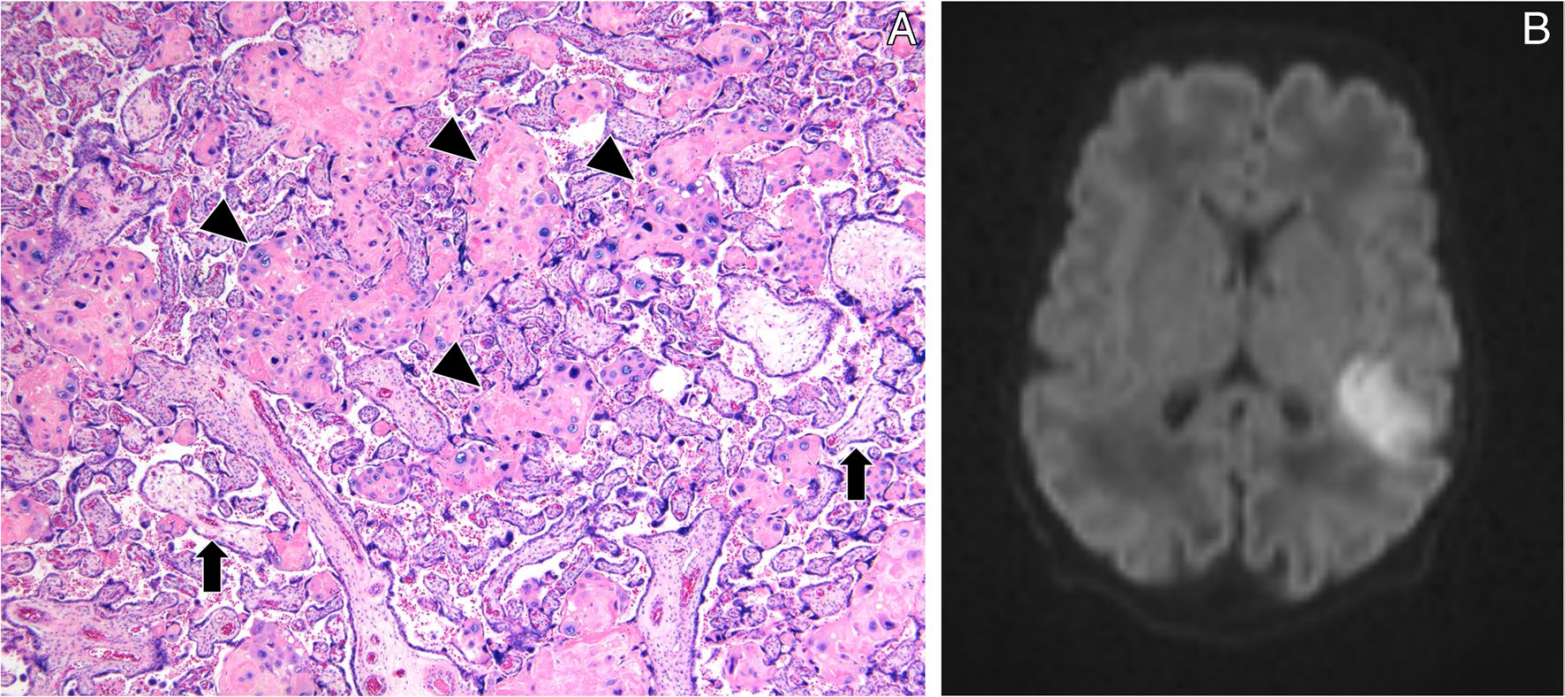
Case P4: in utero crack cocaine exposure. A Increased extravillous trophoblast with atypia is seen (*arrowheads*), together with focal villous edema (*arrows*). B DWI MRI at day 5 demonstrates acute superficial left posterior MCA stroke
